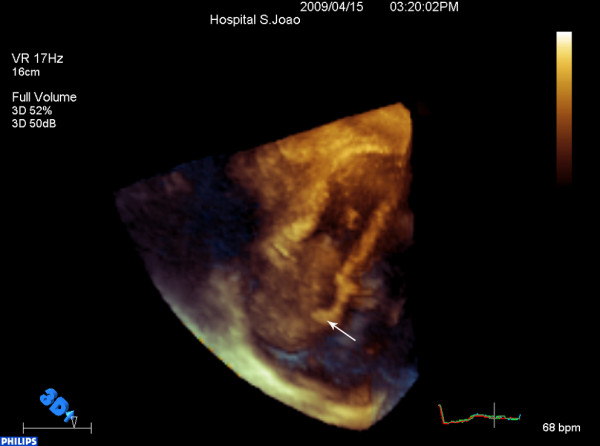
Right atrial mass on 3D-echocardiography. 3 D full volume image reconstruction better defined the mass contour and confirmed its attachment (arrow) in the interatrial septum below the *fossa ovalis*.
